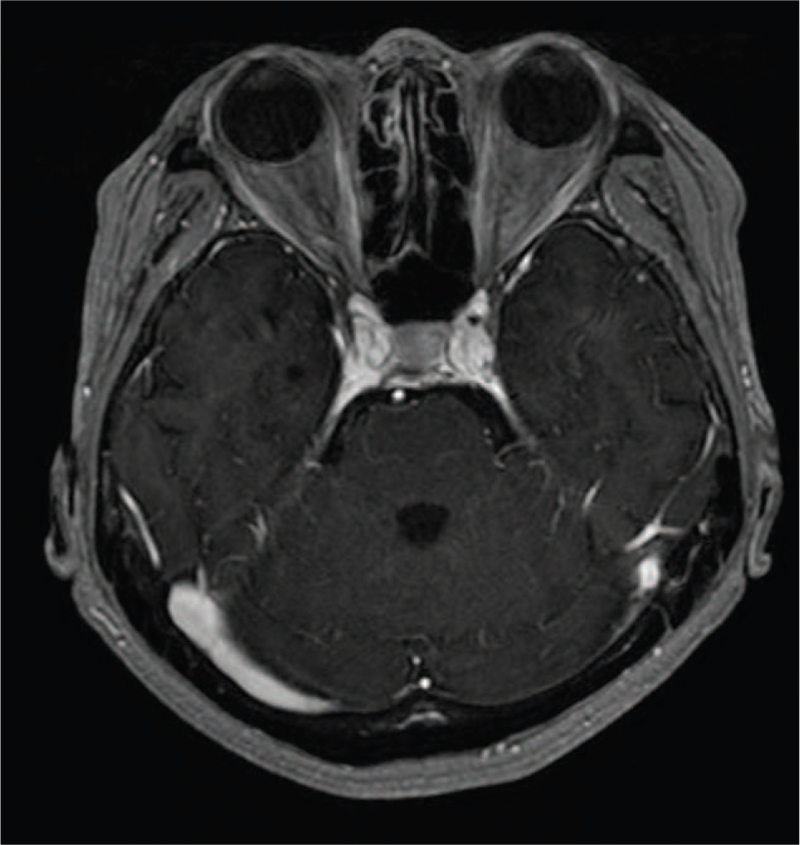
Brain contrast-enhanced magnetic resonance imaging after 3 months after the initiation of lorlatinib shows improvement of the diffuse and linear enhancement along the cerebellar folia.
